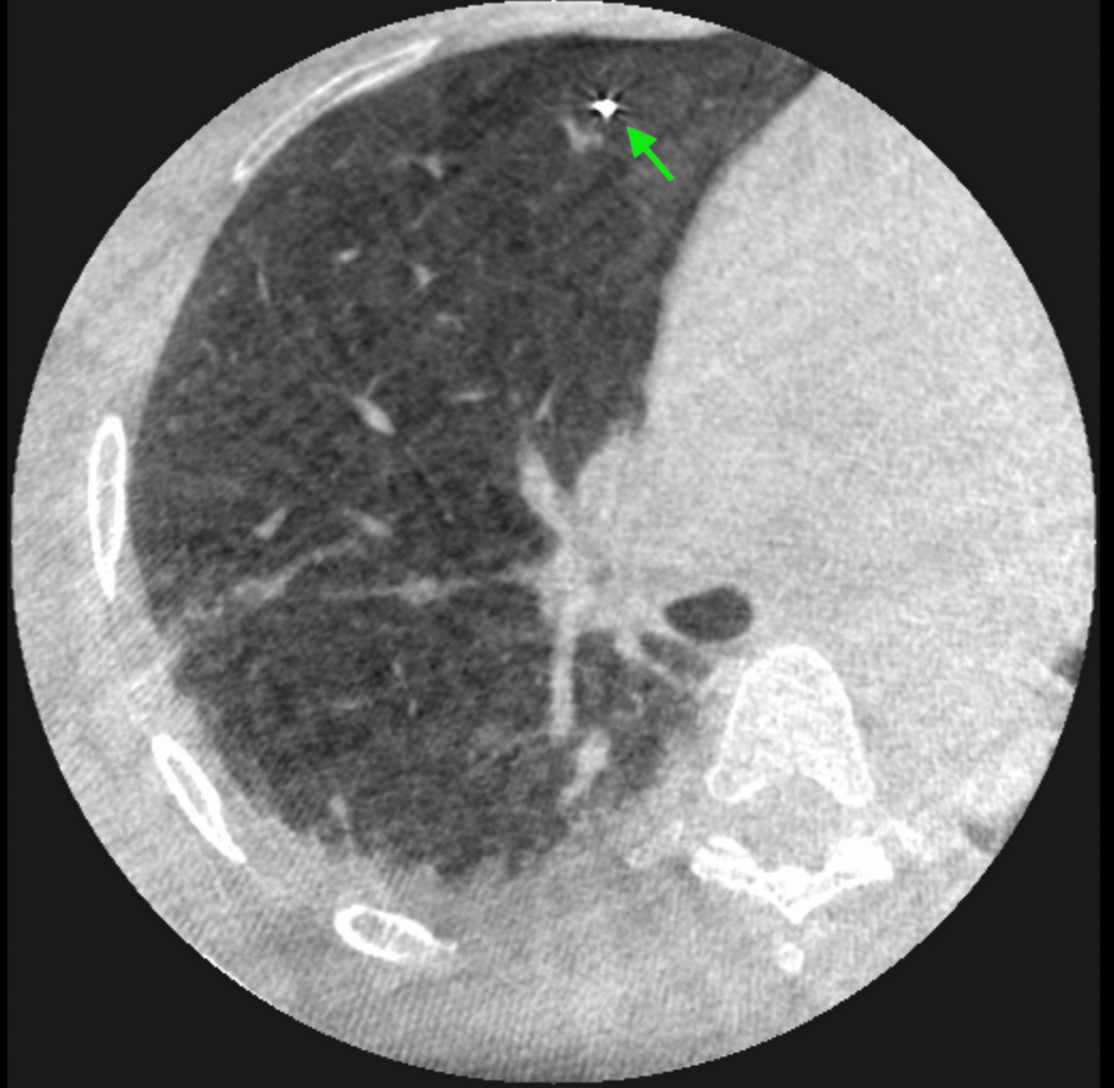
CBCT image of brush catheter adjacent to pleura (axial view). CBCT: cone-beam computed tomography
